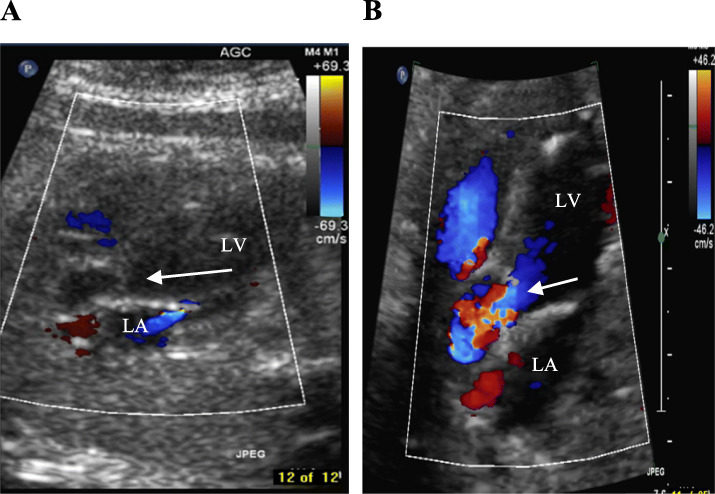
a Before the intervention, no forward flow (arrow) was present through the left ventricular outflow tract and mitral regurgitation was observed by color Doppler. b Immediately after the balloon dilation, echocardiography demonstrated increased forward flow across the aortic valve and disappearance of the mitral regurgitation. LV, left ventricle; LA, left atrium
